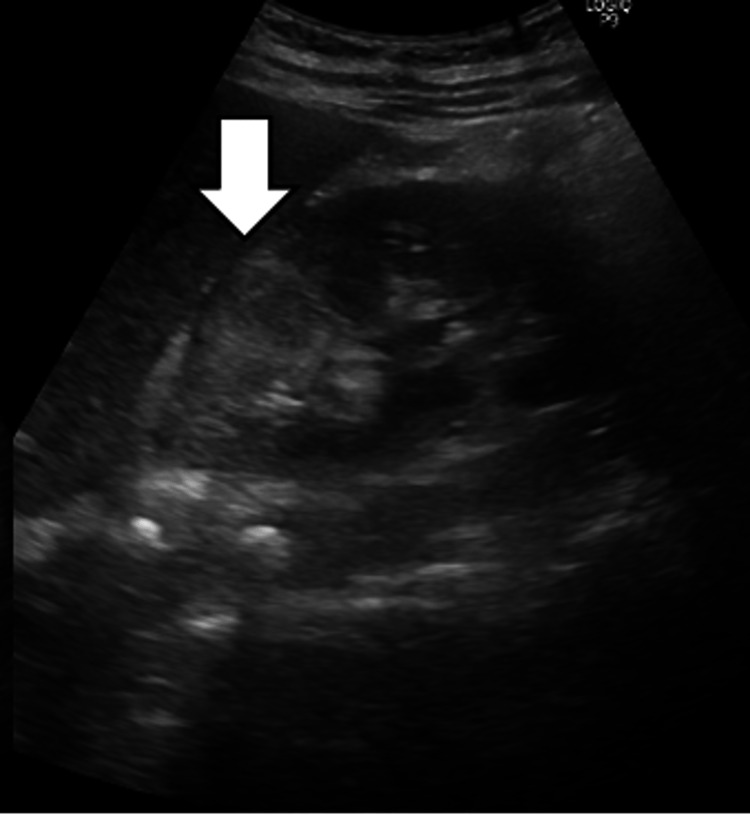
Abdominal ultrasonography showing a nodular shadow (diameter, 32 × 25 mm) on the upper pole of the right kidney
